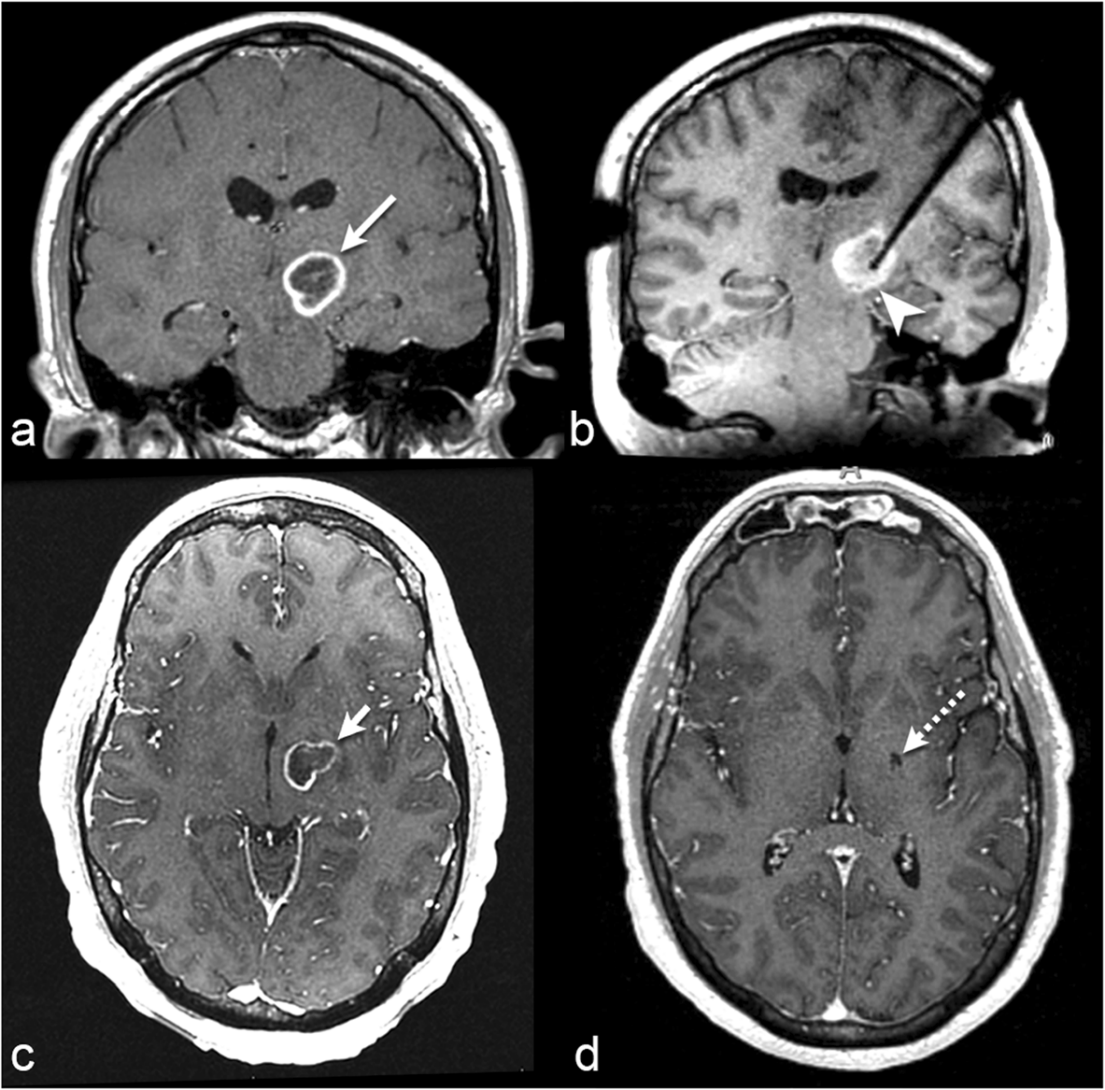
35-year-old with biopsy-proven left thalamic glioblastoma. a Coronal post-contrast T1-weighted MRI before LITT demonstrates a ring-enhancing mass (long arrow). b Coronal intraoperative localizing T1-weighted MRI shows the laser probe within the mass (arrowhead). c Axial post-contrast T1-weighted MRI 2.5 months after LITT shows a mild decrease in size of the mass (short arrow). d Axial post-contrast T1-weighted MRI 4 months after LITT demonstrates complete resolution of the glioblastoma (dashed arrow)
